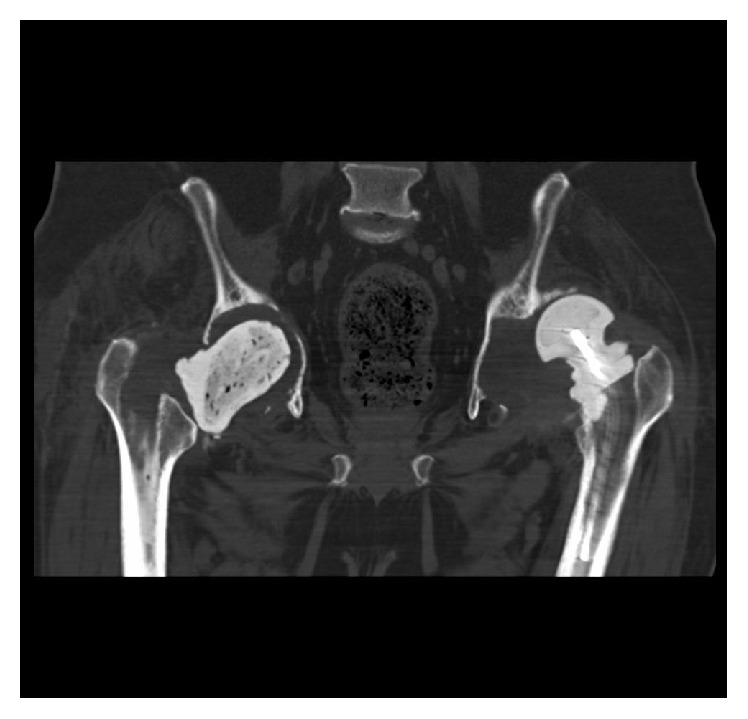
CT scan showing bilateral first-stage revision THR prostheses with right-sided pelvic discontinuity and a severe left-sided posterosuperior acetabular deficiency.
